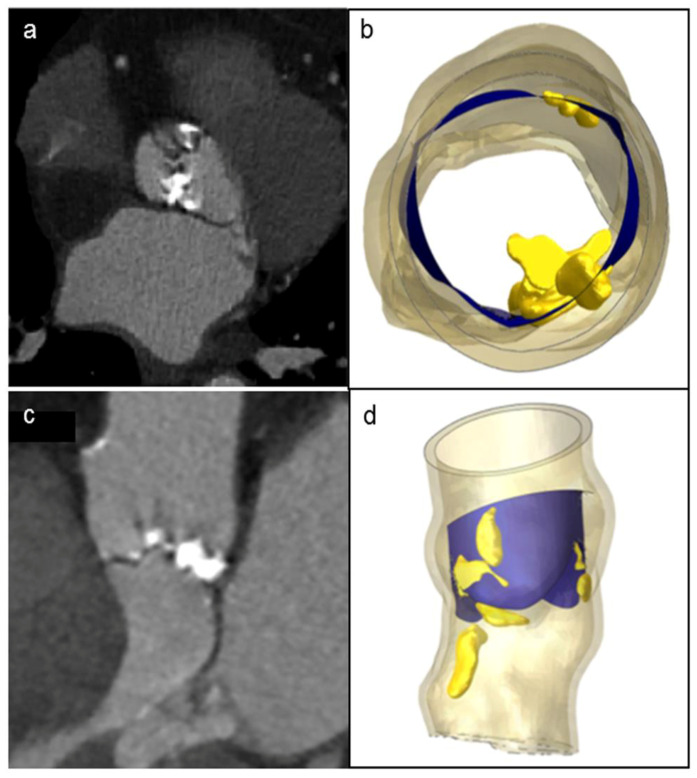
Patient’s preoperative CT scans of severe bulky calcifications attached to aortic valve leaflets and root (a,c), compared to the model reconstruction (b,d).
